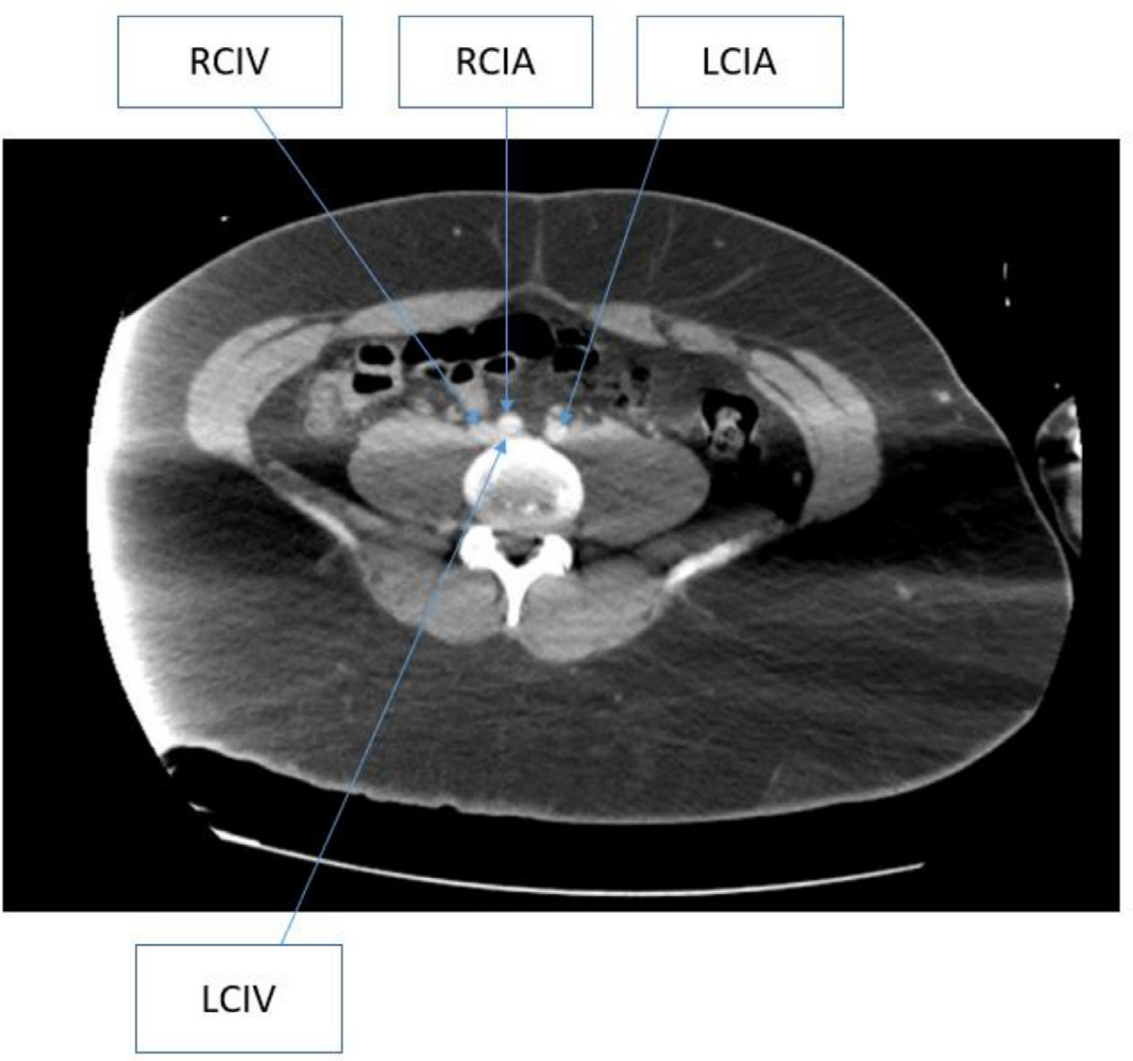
Contrast-enhanced CT of the abdomen and pelvis showed compression of the left common iliac vein by the right common iliac artery.
